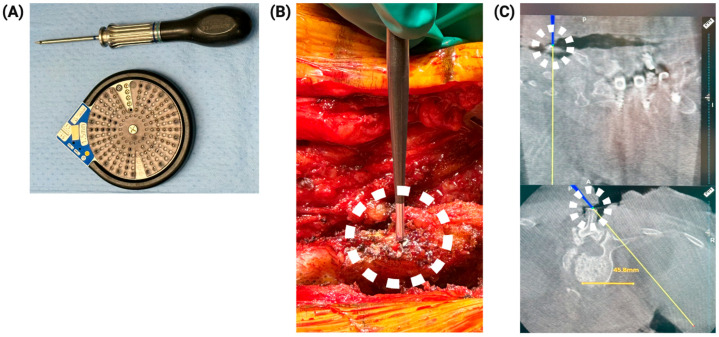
Accuracy confirmation using navigation: The Medtronic Stealth Navigation System accuracy is confirmed with internal fiducials. (A) Shows the Stryker screws used. (B) Displays the navigated instrument making contact with the Stryker screw on the patient’s spinous process in the surgical field. (C) Displays the navigated instrument making contact with the Stryker screw (metallic hyper-density) on the intraoperatively obtained CT images. The accuracy demonstrated here is excellent.
